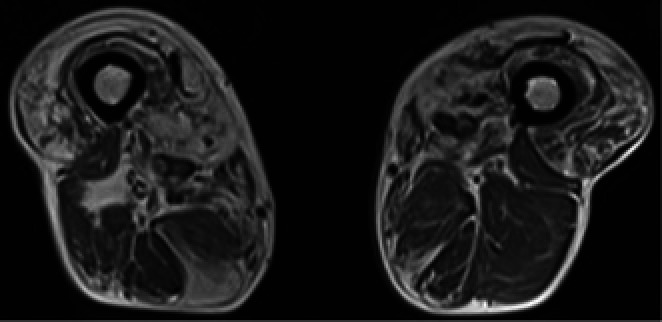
T1‐weigthed MRI image of both thighs showing anterior distal fatty infiltration.
